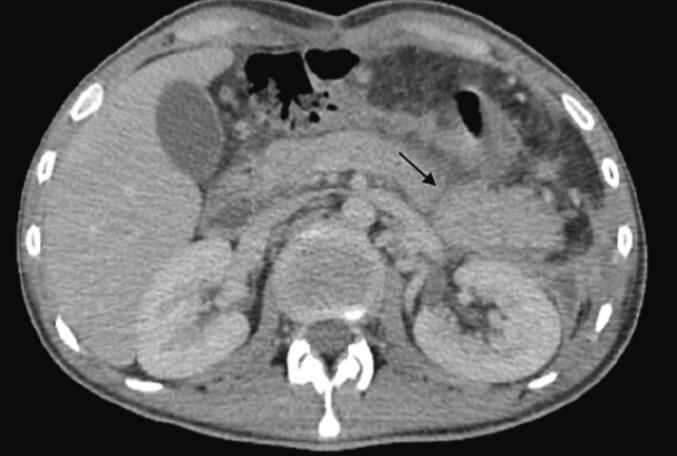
Axial CT section taken on day 10 showing fracture line of the tail of the pancreas.
